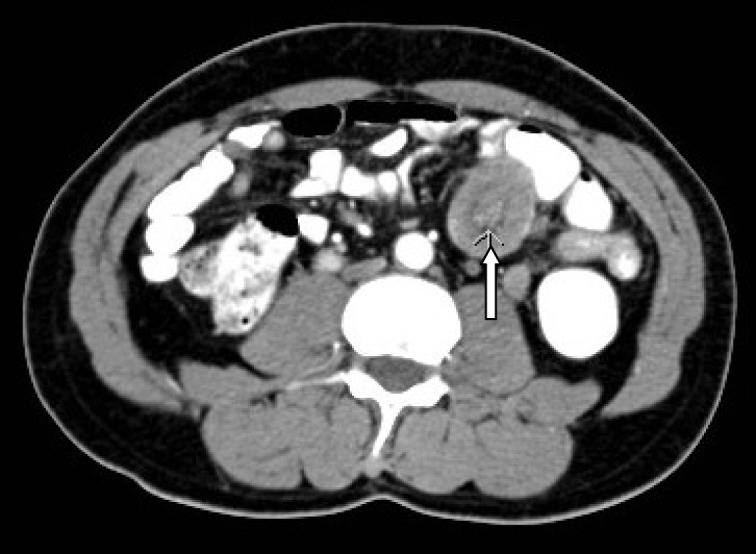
CT-scan showing small intestines intussusceptions secondary to a metastasizing malignant melanoma.
